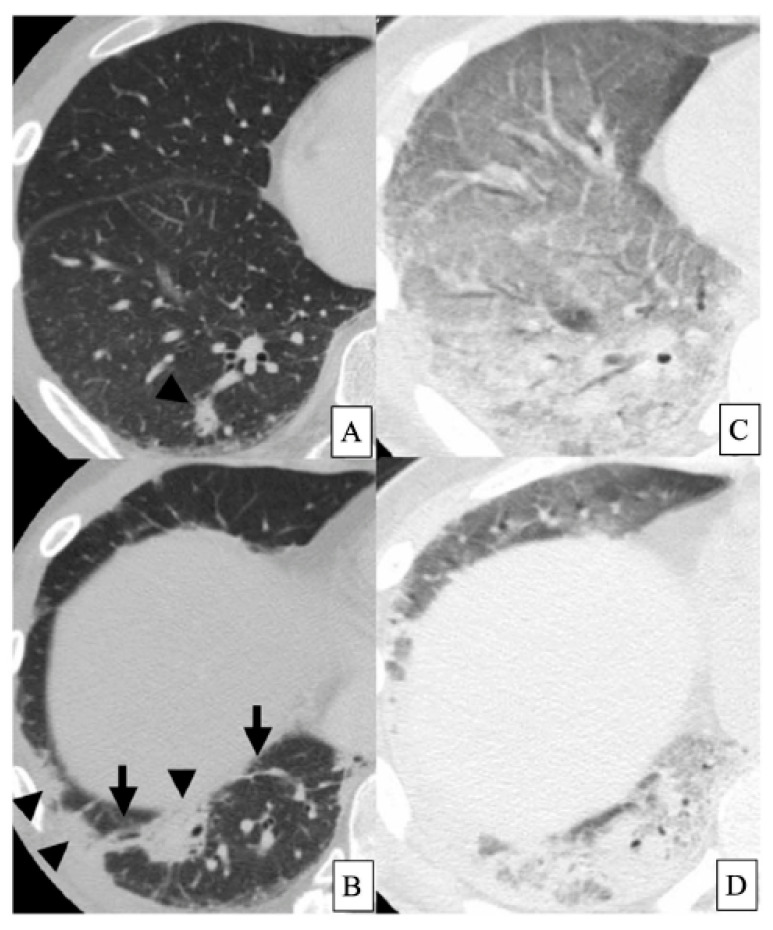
HRCT findings of the lungs in a patient with DM-ILD with anti-MDA5 antibody. A forty-four-year-old male patient with DM-ILD was positive for anti-MDA5 antibody. At diagnosis, peripheral and peribronchovascular consolidations are observed (arrowheads). Interlobular septal thickening and non-septal linear or plate-like opacities are also seen (arrows) ((A,B), from Tanizawa et al. [92] with permission). Despite treatments for 6 weeks, severe respiratory failure developed, requiring mechanical ventilation. Diffuse GGA and consolidation with air bronchograms are extended in the whole lungs (C,D) [92]. Surveillance at this point revealed no evidence of infection. The patient died of respiratory failure one week later. Abbreviations: HRCT, high-resolution computed tomography; DM, dermatomyositis; ILD, interstitial lung disease; MDA, anti-melanoma differentiation-associated gene; GGA, ground-glass attenuation.
